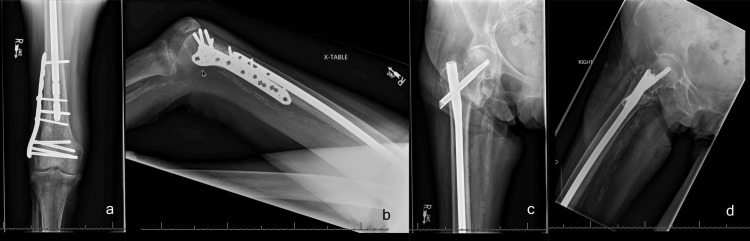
One-year postoperative X-rays (a) Anteroposterior view: distal; (b) lateral view: distal; (c) anteroposterior view: proximal; (d) lateral view: proximal.
